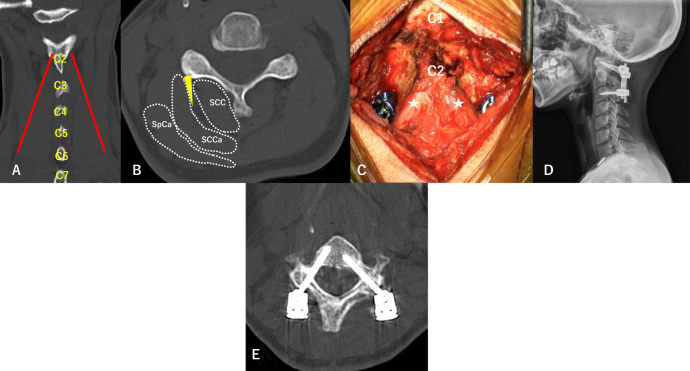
Preoperative, intraoperative, and Postoperative findings during posterior C1–3 fixation. (A) Preoperative CT demonstrating the anatomical course of the semispinalis cervicis (SSC) muscle (red line). (B) Preoperative CT demonstrating the anatomical structure of the posterior cervical muscle and fat plane (yellow area) at the C3 level. SSC, Semispinalis cervicis muscle; SCCa, semispinalis capitis; SpCa, splenius capitis. (C) Intraoperative photograph showing the preserved SSC (★) during screw placement; screws placed via a lateral trajectory beneath SSC retraction. (D) Postoperative X-ray demonstrating appropriate alignment and secure placement of the obliquely inserted cervical pedicle screws. (E) Postoperative CT demonstrating that pedicle screws were inserted nicely and obliquely.
